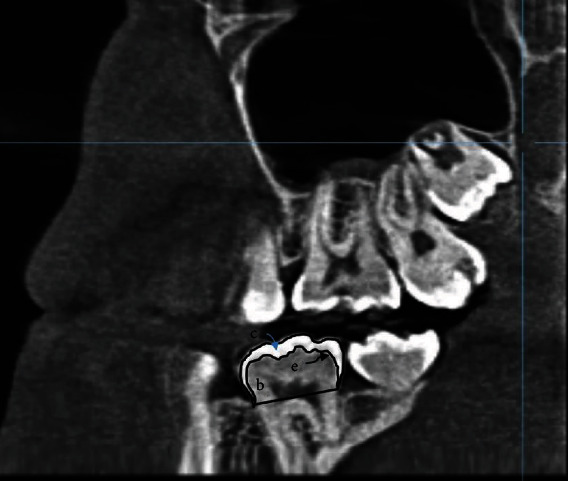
Relative enamel thickness (RET) measurement on CBCT image. *c* = area of enamel cap in mm^2^; *e* = length of DEJ in mm; *b* = area of dentin under DEJ in mm^2^.
